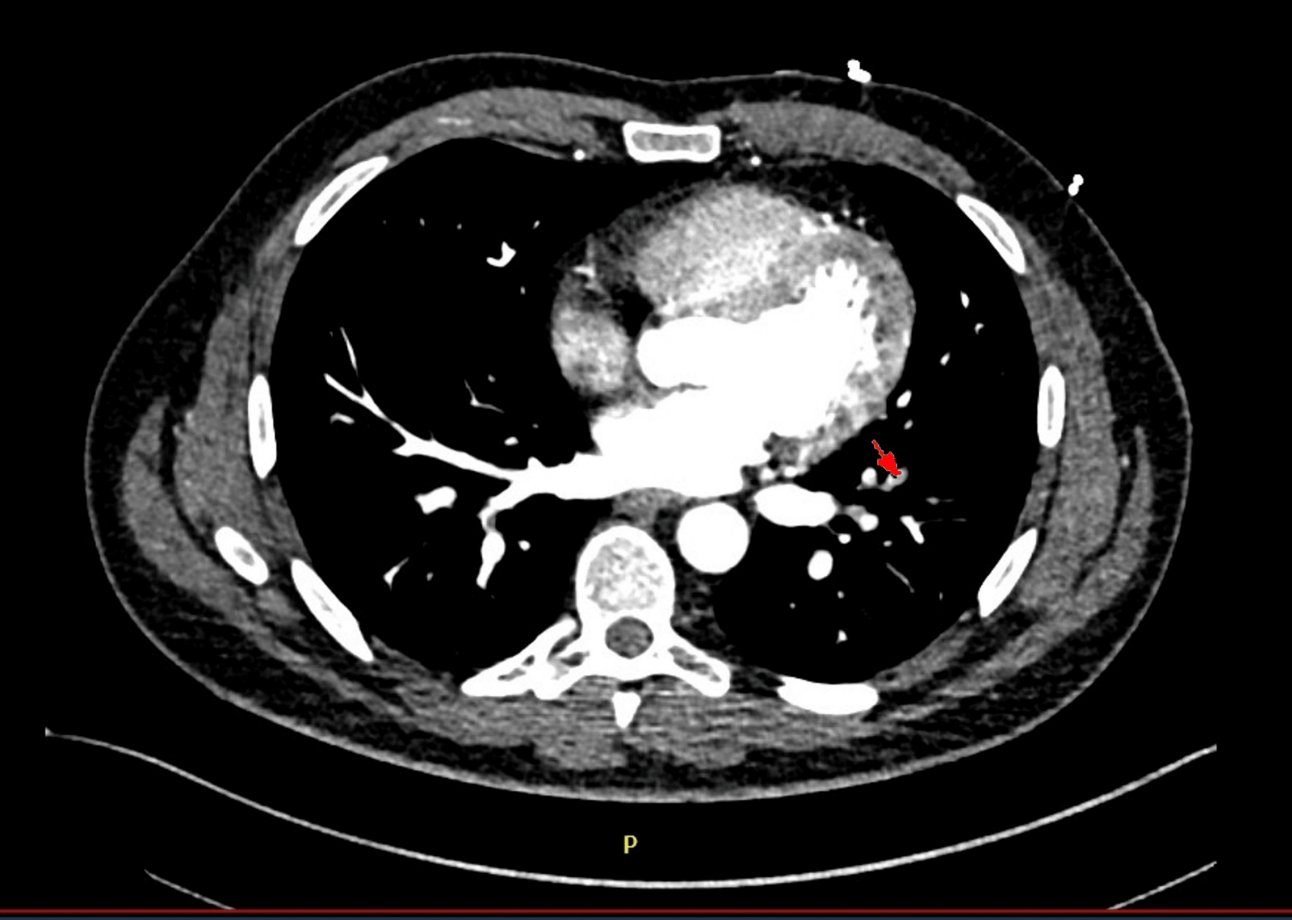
CT pulmonary angiogram Arrow pointing towards a low-volume pulmonary embolism in the distal segment of the left lower lobe.
